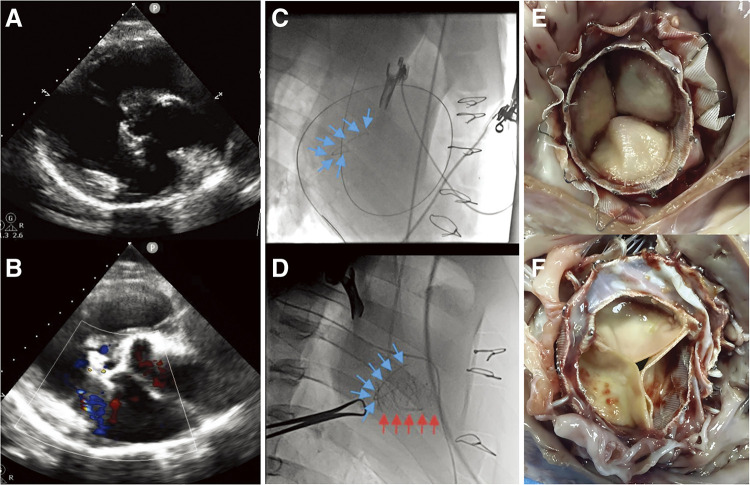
Postoperative Management. (A and B) Echocardiography: Appropriate position of the bioprosthesis, with mild central tricuspid regurgitation but no perivalvular leakage. (C and D) Fluoroscopy: transjugular tricuspid valved stent implantation. Tricuspid annulus prosthesis (blue arrows), valved stent (red arrows). (E and F) Pathology: The valved stent were well positioned on gross examination. Part of the annulus was covered by endothelial tissue. Pathology did not show any significant thrombosis, incrustation or calcified nodules in the tissue between the stent and the original TA.
